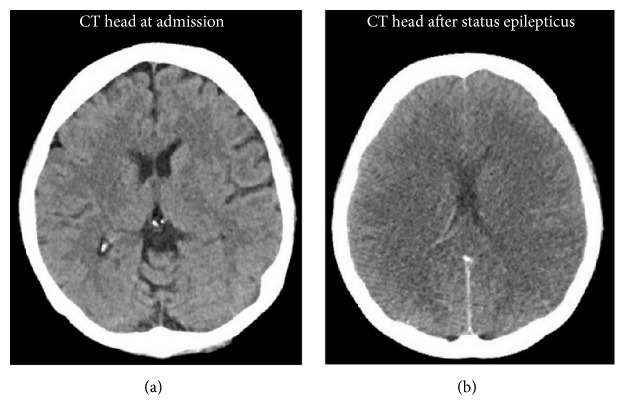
Computed tomography of the head at admission and at onset of status epilepticus. Computed tomography (CT) of the head (a) showed no remarkable findings on the day of admission and (b) showed diffused brain swelling consistent with cerebral edema on 12th day.
